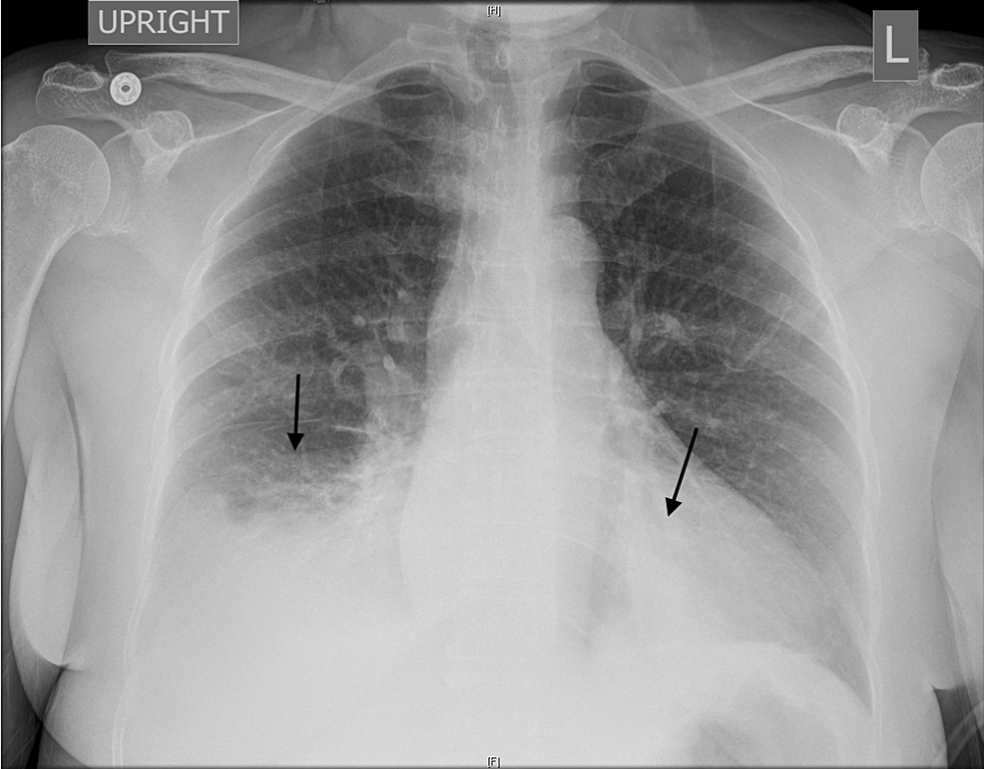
Chest radiography on the second presentation The left arrow depicts right basilar opacity, and the right arrow depicts retrocardiac opacity.
